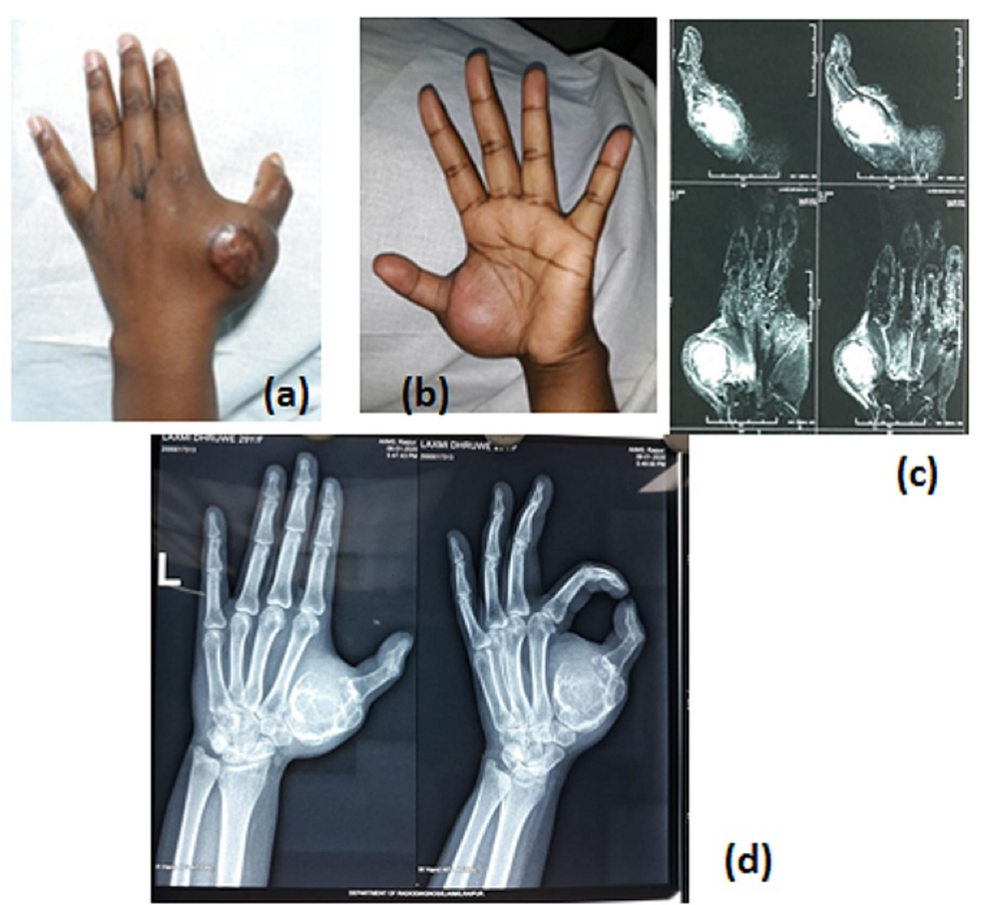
(a, b) Clinical picture of the left thumb, (c) MRI, and (d) radiograph showing expansile osteolytic lesion of the first metacarpal
